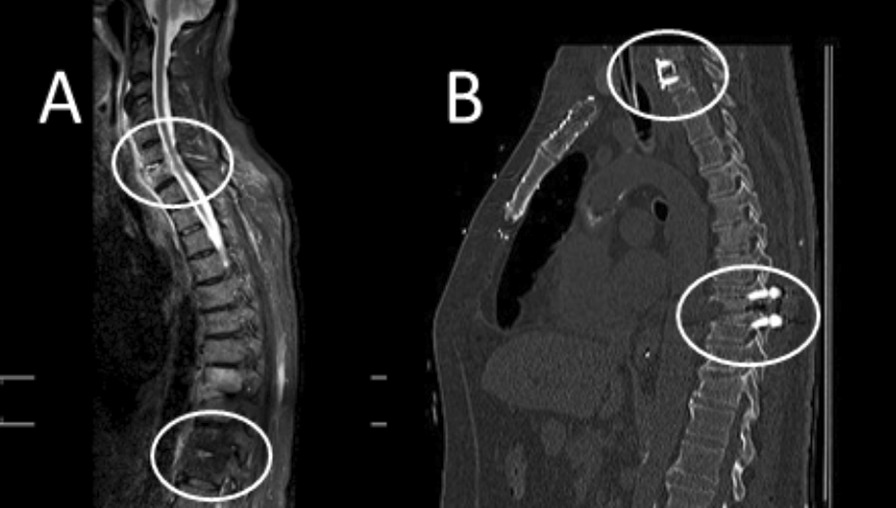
MRI image showing ML PSD at cervical und thoracic spine, A before surgery, B after surgery (postoperative CT scan)
